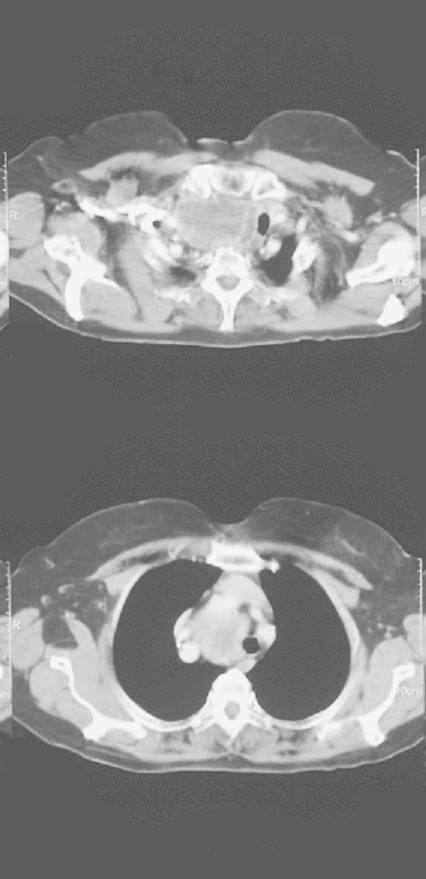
CT scan large mass in the right lobe of thyroid grand invasive to the upper mediastinum.
